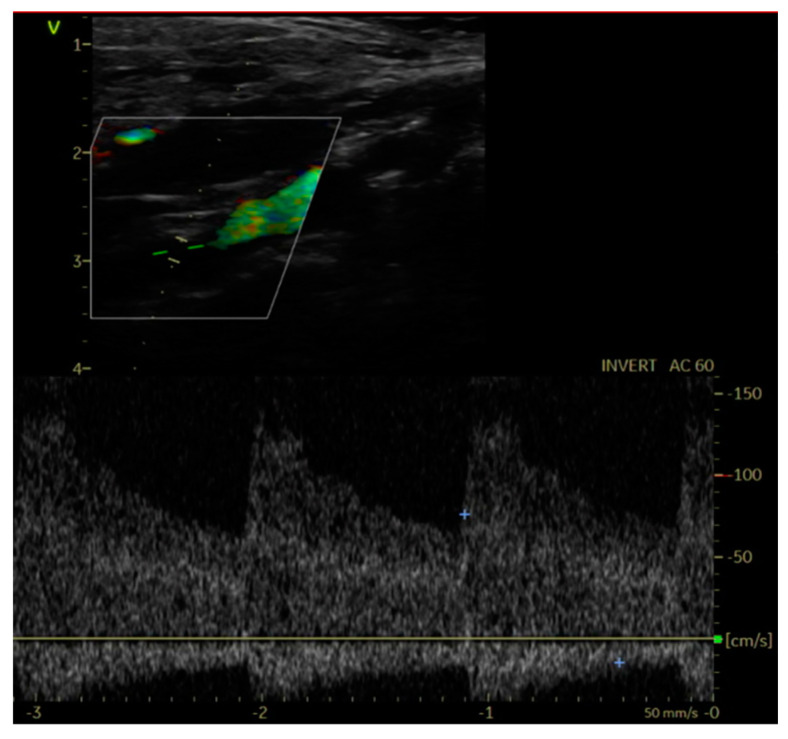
High−velocity flow in FMD stenosis (PW Doppler).
